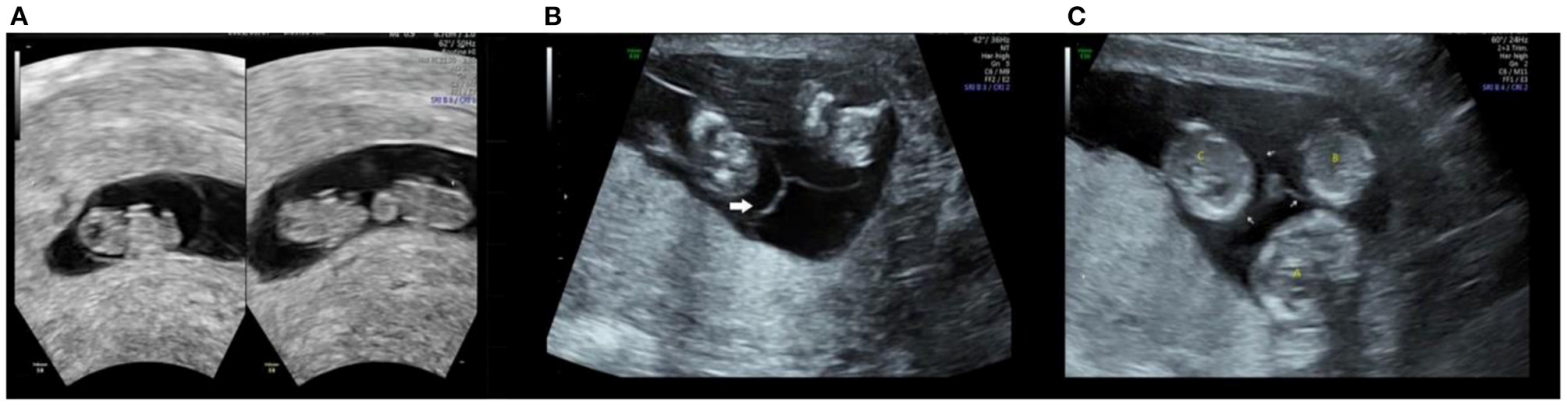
Ultrasound image during the first trimester. At the 59th days of pregnancy, ultrasonography shows one gestational sac with three yolk sacs (A). At 13 0/7 weeks of gestation, fetuses (A–C) are separated by intertwin membranes (arrow).
